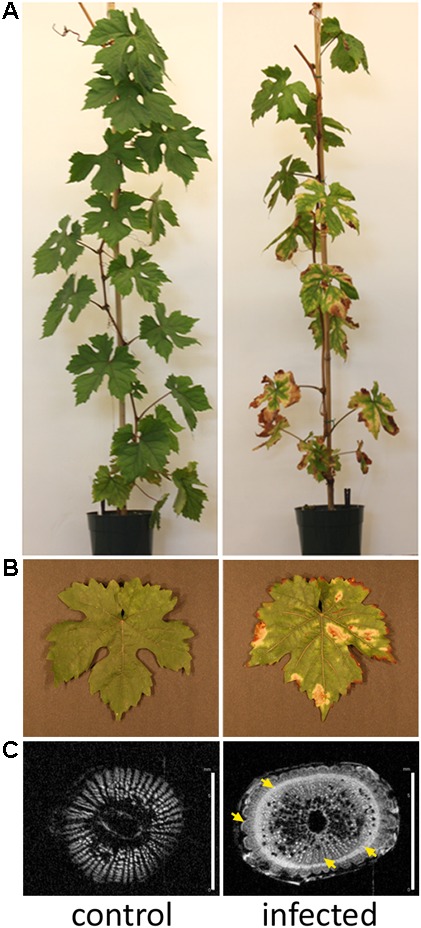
Grapevines developing Pierce’s disease in controlled inoculations. (A) Twelve weeks after inoculation of *X. fastidiosa*, grapevines already displayed Pierce’s disease symptoms as leaf scorching, match stick petioles from fallen leaves, and darkened patches on stems. (B) Detail of leaf showing scorching symptoms. (C) Nuclear magnetic resonance imaging of representative transversal cuts of stems exhibiting intensive secondary wall deposition in infected vines. Yellow arrows point to electron-denser material more abundant in infected samples. Scale bar is 6 mm for both images.
